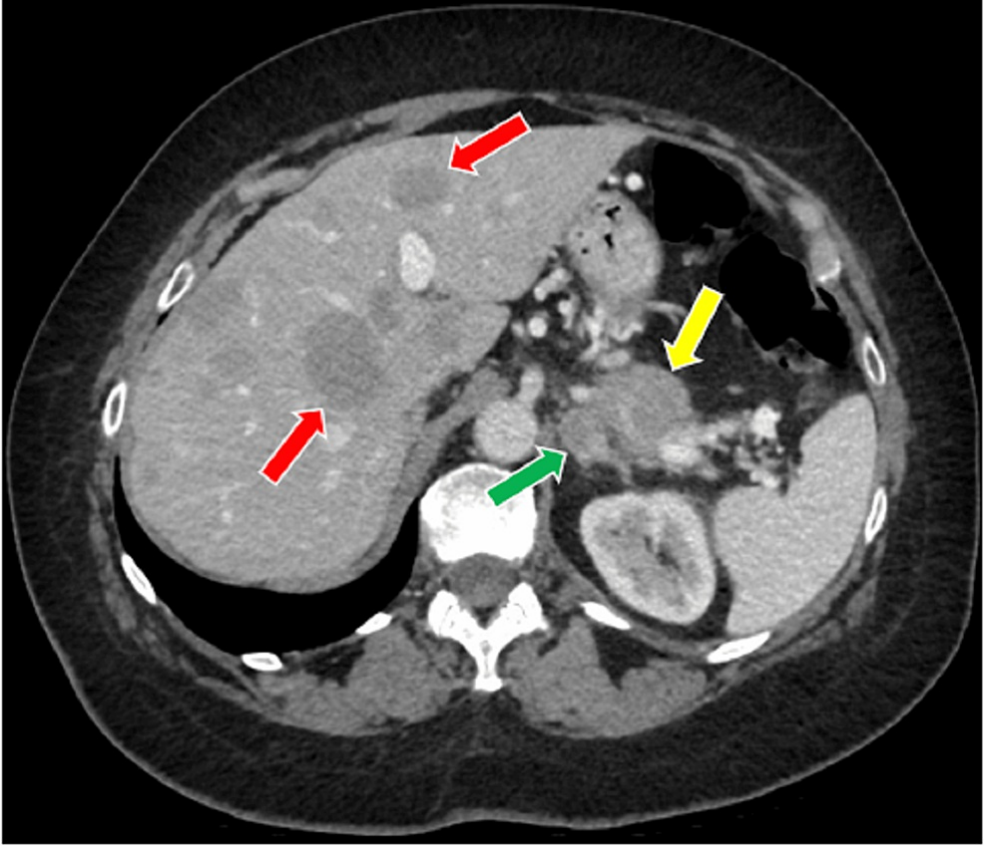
CT axial plane image of left adrenal mass (green arrow) adjacent to primary pancreatic mass (yellow arrow) and hepatic metastases (red arrows)
